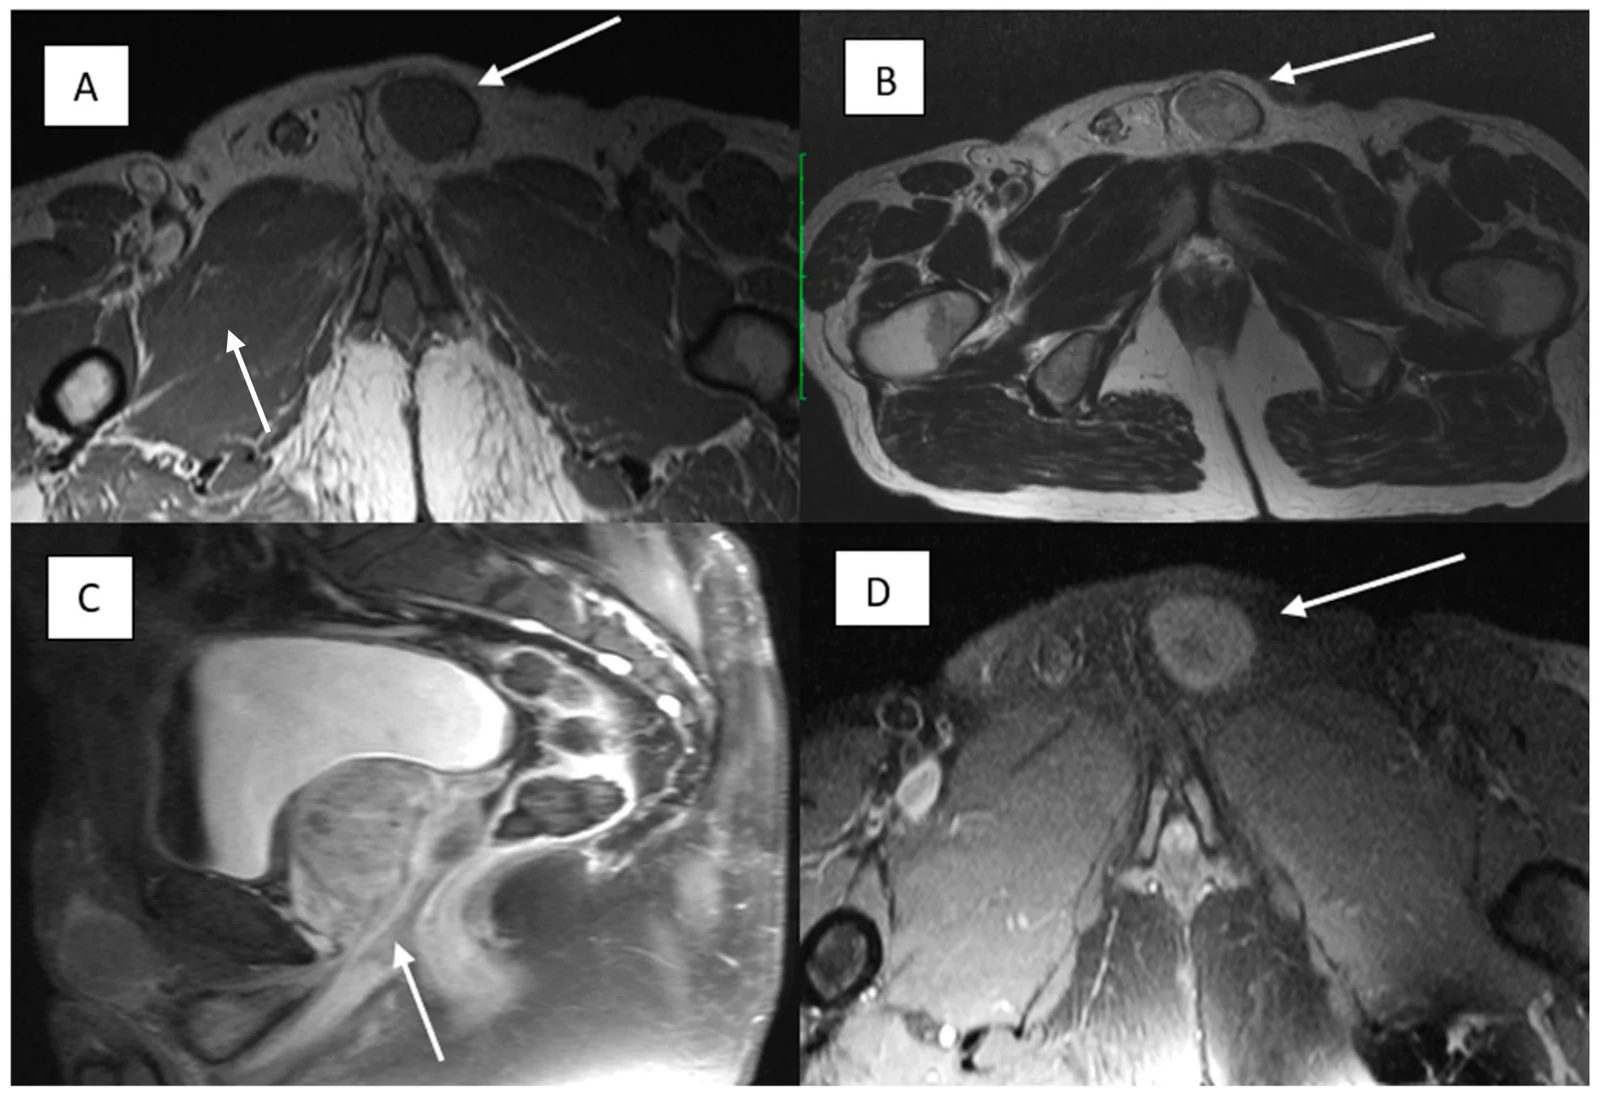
Magnetic resonance imaging of the left cryptorchid testis. The white arrow identifies the left undescended (cryptorchid) testis. (A,B) Native axial T1- and T2-weighted images demonstrating a relatively well-circumscribed intratesticular lesion with a heterogeneous pseudocystic appearance. (C,D) Post-contrast sagittal and axial T1-weighted images showing enhancement of the solid component, whereas the cystic component shows no contrast enhancement.
